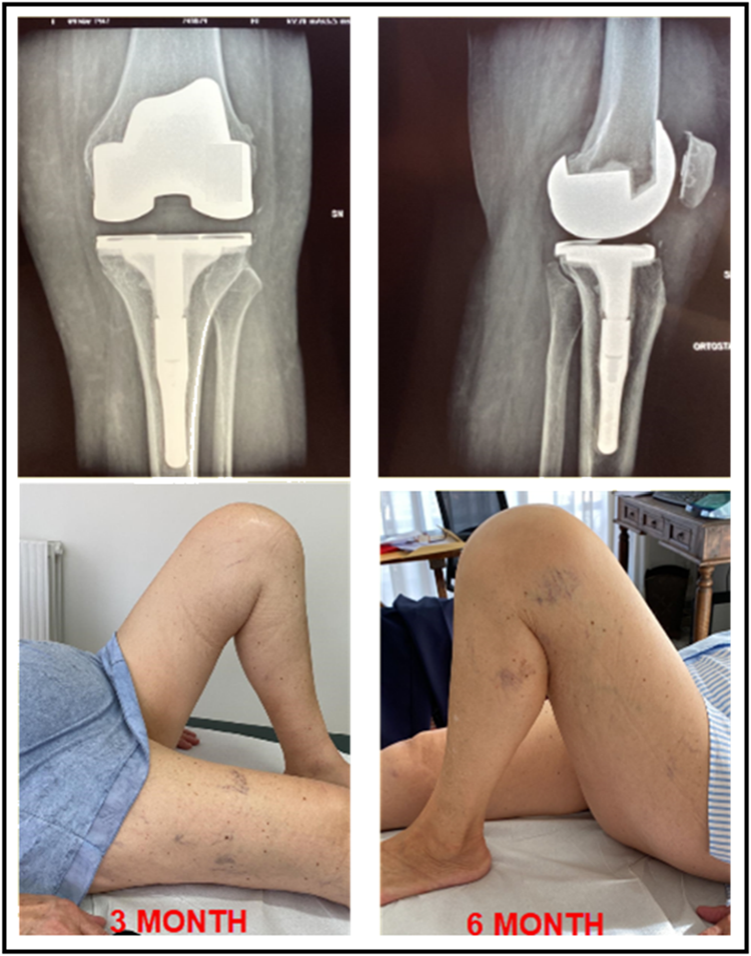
Post‐op radiographs and 3‐ and 6‐month post‐op photographs.
